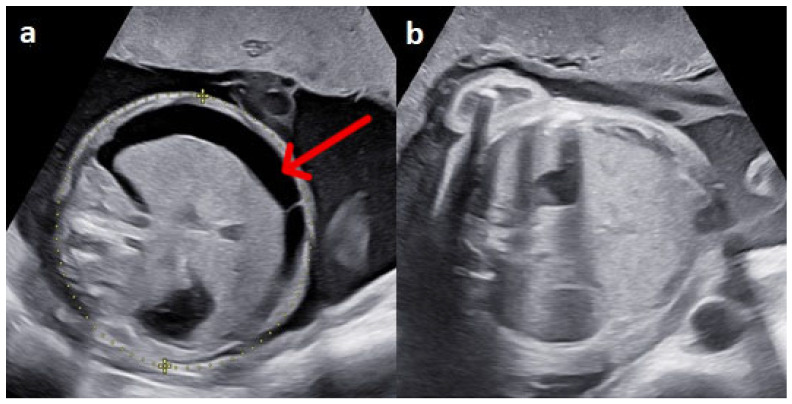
Resolution of fetal ascites after intrauterine transfusions (IUTs). Transverse ultrasound view of the fetal abdomen. (a) Massive fetal ascites (red arrow) at 24 weeks of gestation, at the time of hemolytic disease of the fetus diagnosis. (b) Complete resolution of ascites at 27 weeks of gestation following three IUTs.
